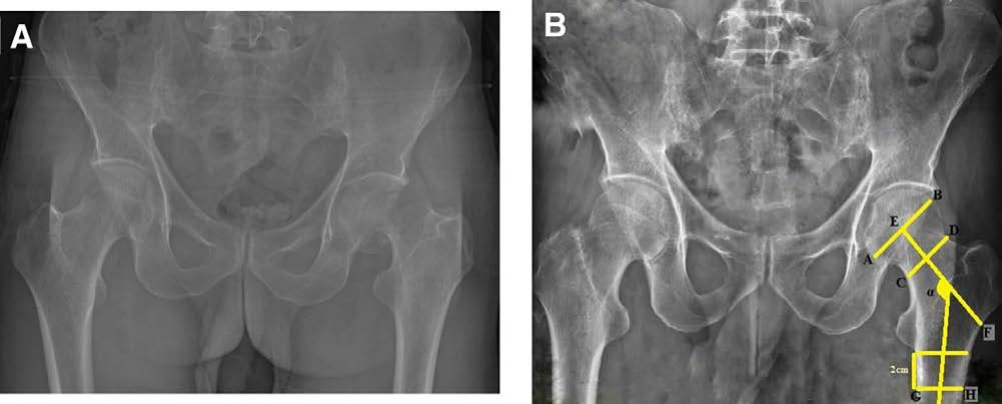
Anteroposterior radiograph of the pelvis shows the neck shaft angle (α), femoral head diameter (line AB), femoral neck diameter (line CD), femoral neck length (line EF), femoral shaft diameter (line GH) (B) and post-traumatic fracture radiograph of the same patient (A).
